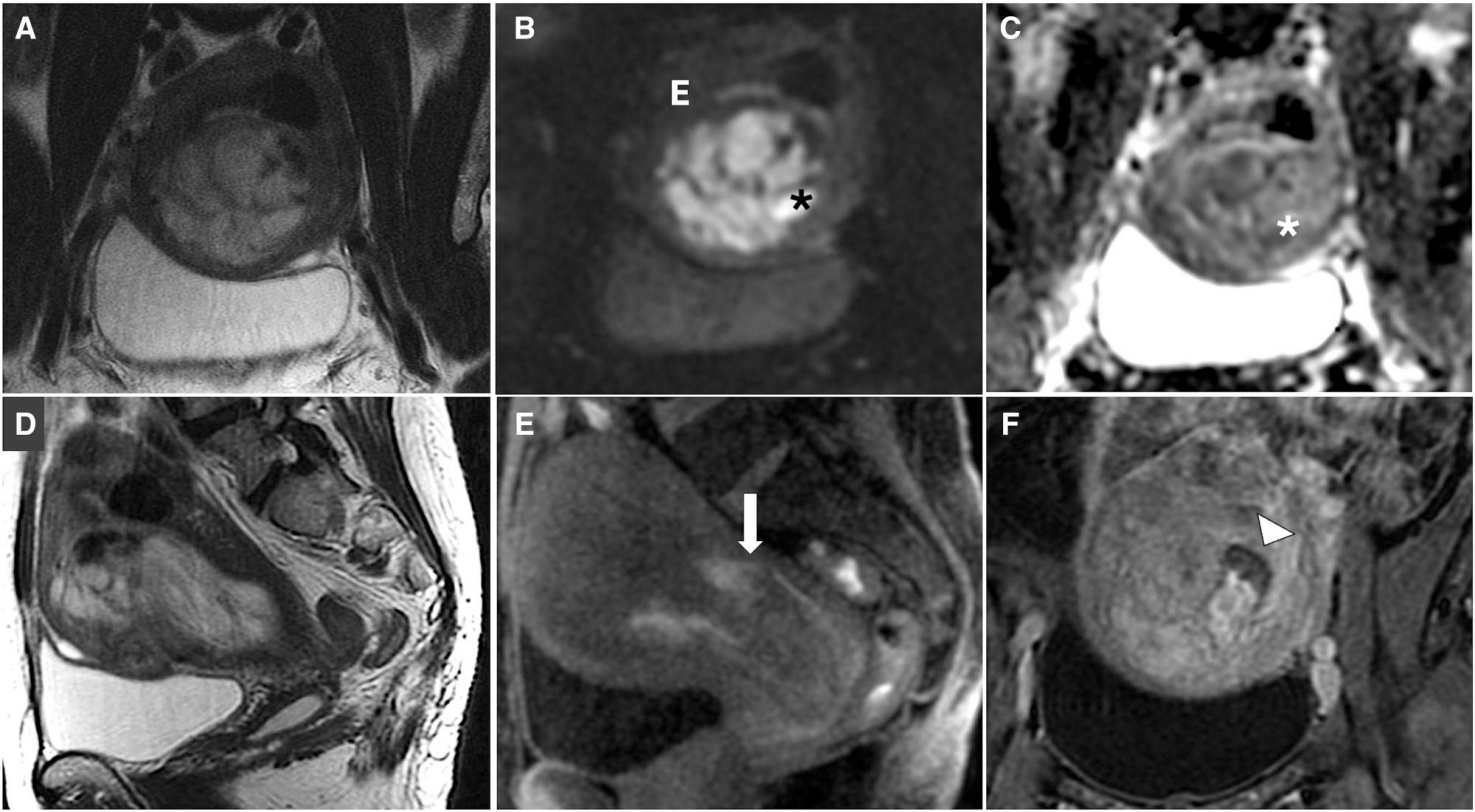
Leiomyosarcoma. MR images show a large, irregular lesion with high SI on T2-WI (A, D), with areas of viable tissue (asterisk) demonstrating higher SI on DWI (B) compared with the endometrium (E) and corresponding low SI on ADC map (C). Heterogeneous appearance on T1-WI (E) and postcontrast images (F) is due to the presence of haemorrhage (arrow) and necrosis (arrowhead). Abbreviations: SI = signal intensity; WI = weighted imaging.
